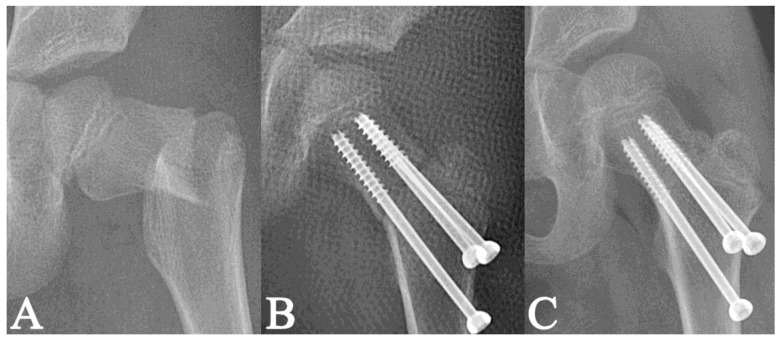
Radiographs of a 5-year-old girl with a displaced Delbet–Colonna type II fracture [15] (A); radiographs after closed reduction and internal fixation with 3 cannulated screws (B); the radiological healing at 3.9 months post-surgery (C).
